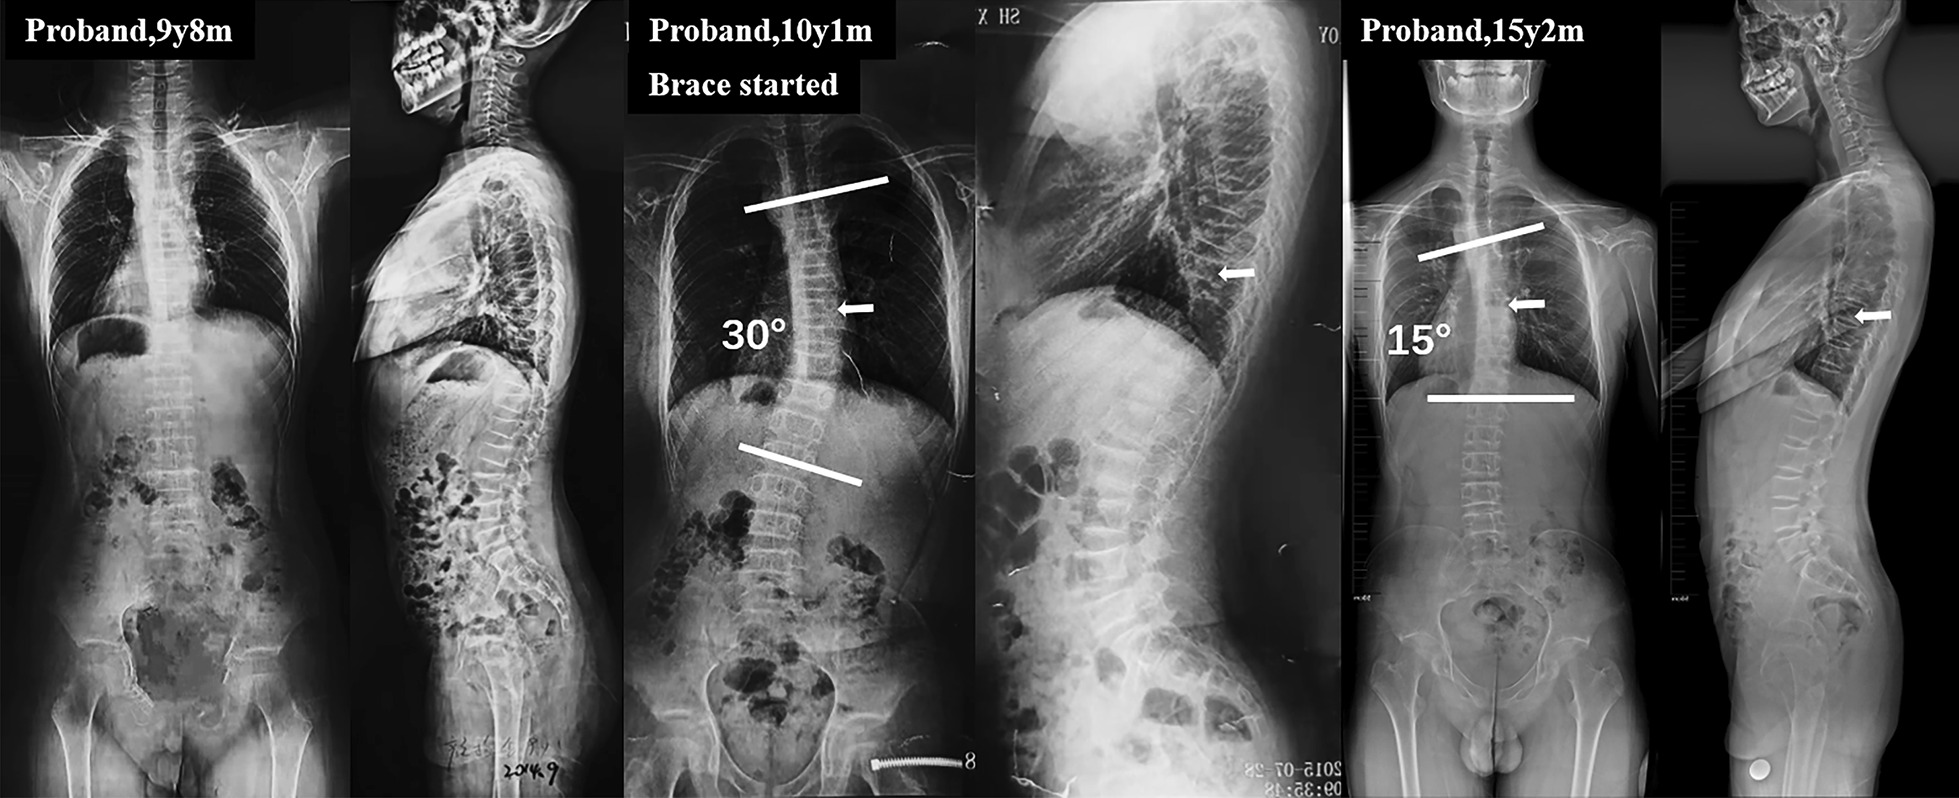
Standing anteroposterior and lateral spinal radiographs of the proband. a Spinal radiographs showed lumbar compression fracture. b The proband exhibits the phenotype of scoliosis 3 months after lumbar compression fracture. c The cobb angle decreased to 15° and the proband reached skeletally mature after 5 years of brace treatment. White arrows indicated the apical vertebra
